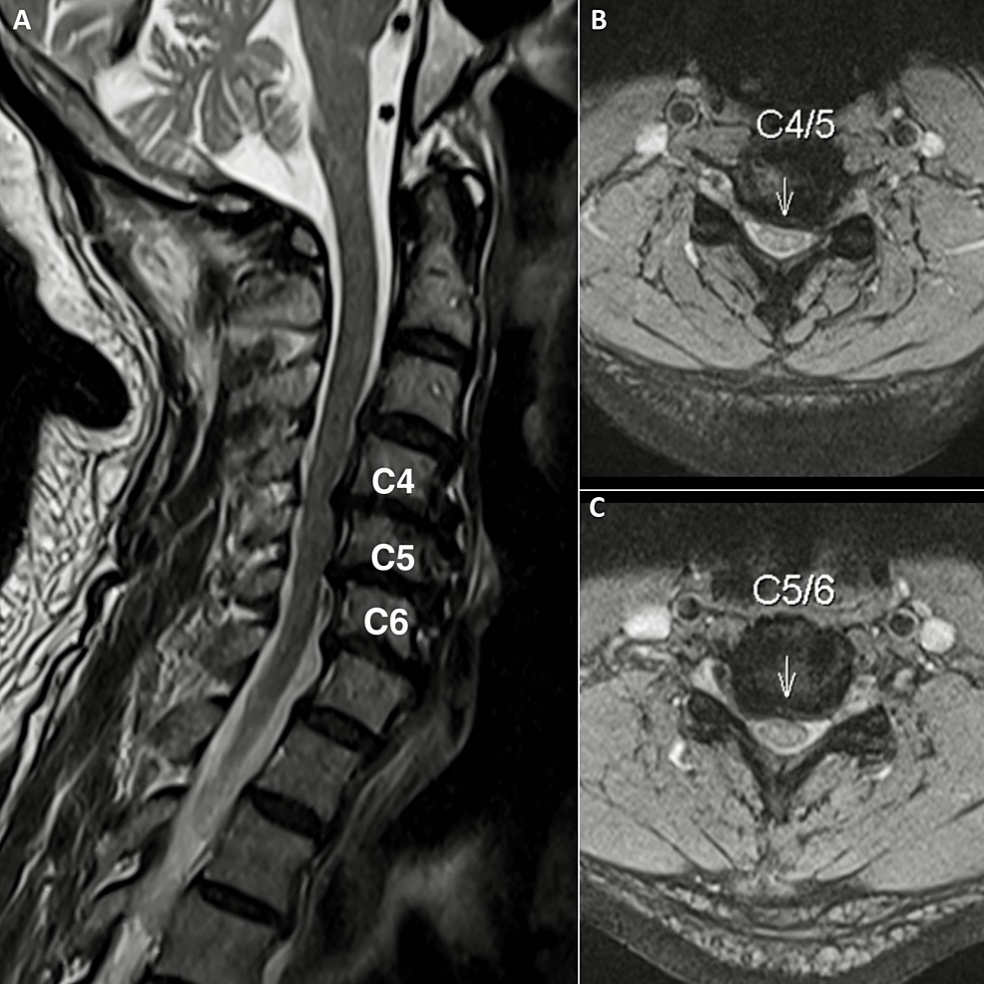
Magnetic resonance imaging of the cervical spine A) Cervical spondylosis with diffuse disc desiccation and decreased disc height at C4/5, C5/6, and C6/7 levels. B) Spinal stenosis at C4/5 and C5/6 levels with cord impingement but no abnormal cord signal.
